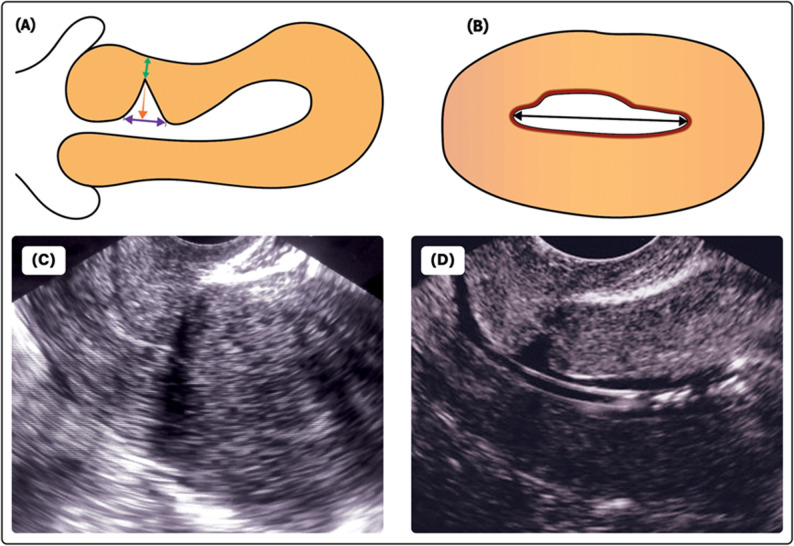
Uterine niche measurements used in the study. A Sagittal plane demonstrating niche depth (red arrow), niche length (blue arrow), and residual myometrial thickness (RMT; green arrow). B Transverse plane demonstrating niche width (black arrow). C Transvaginal ultrasound image of uterine cesarean scar niche. D Saline-infusion sonohysterography image of the same case
